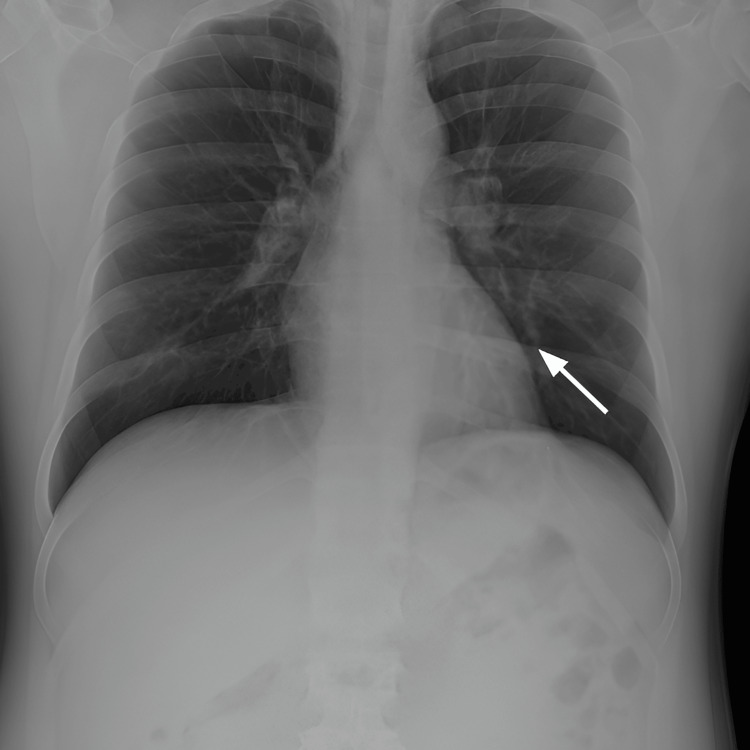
Posteroanterior chest radiograph Posteroanterior chest radiograph demonstrating right parahilar lymphadenopathy (white arrow), consistent with mediastinal involvement. This radiographic finding supports pulmonary involvement in disseminated coccidioidomycosis.
